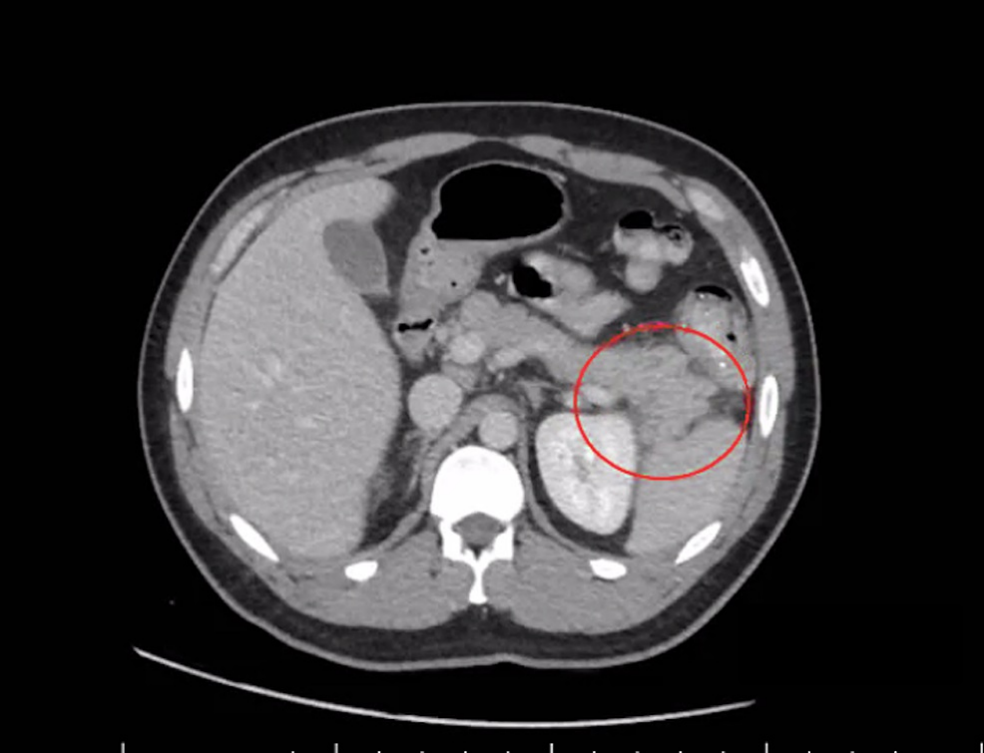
APCT scan with contrast showing mild pancreatitis (red circle) involving the pancreatic tail APCT: abdomen/pelvis computed tomography.
